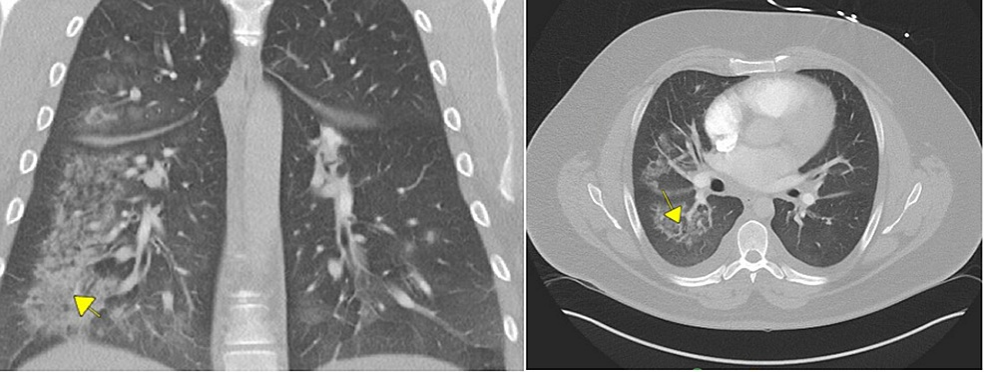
CT chest showing bibasilar airspace opacities, predominantly right-sided and prominent left ventricle enlargement (sagittal, coronal section). CT: computed tomography
